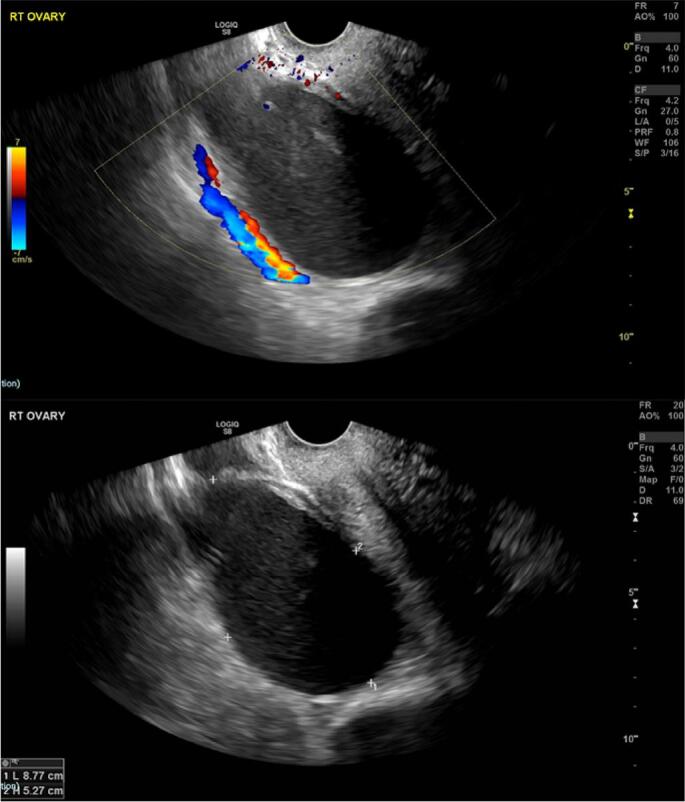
Follow up antenatal ultrasound which showed an increase in size to 9 cm at 4 month interval scan.
